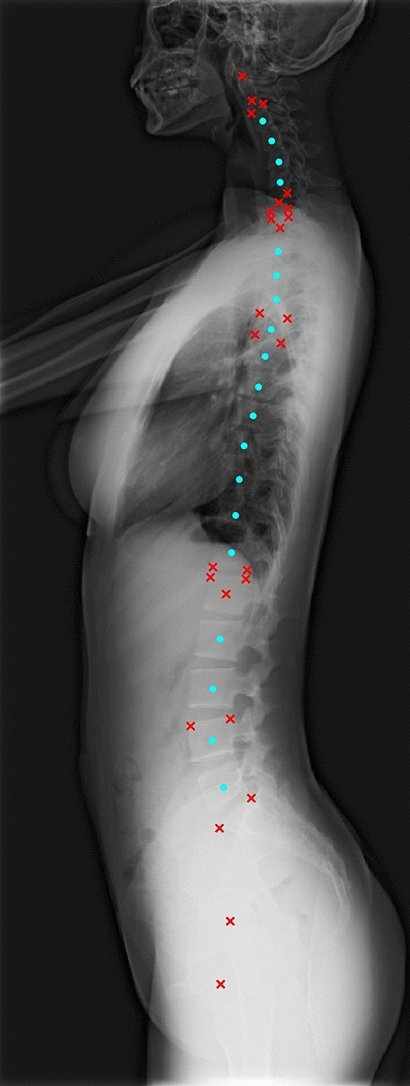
Annotated anatomic landmarks. 45 anatomic landmarks were annotated on a whole-spine lateral radiograph. 26 landmarks related to spinopelvic parameters are highlighted by crosses (in red); the other landmarks, which are at vertebral centres and can be used to determine the spinal curve, are highlighted by solid circles (in cyan). Detailed descriptions for each landmark are presented in the Methods section.
